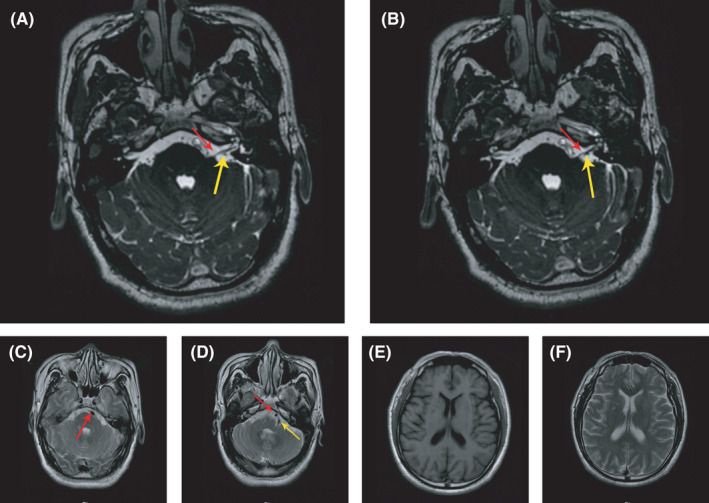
(A and B) Facial nerve MRI shows branching vessels adjacent to the left facial nerve. The red arrow shows the left facial nerve, and the yellow arrow shows the branching vessel. (C and D) Magnetic resonance angiography (MRA) shows tortuous expansion of vertebrobasilar artery. The red arrow shows the tortuous dilated basilar artery, and the yellow arrow shows the tortuous left vertebral artery. (E and F) T1‐weighted and T2‐weighted brain MRI show no obvious hyperintensity of the basal ganglia
